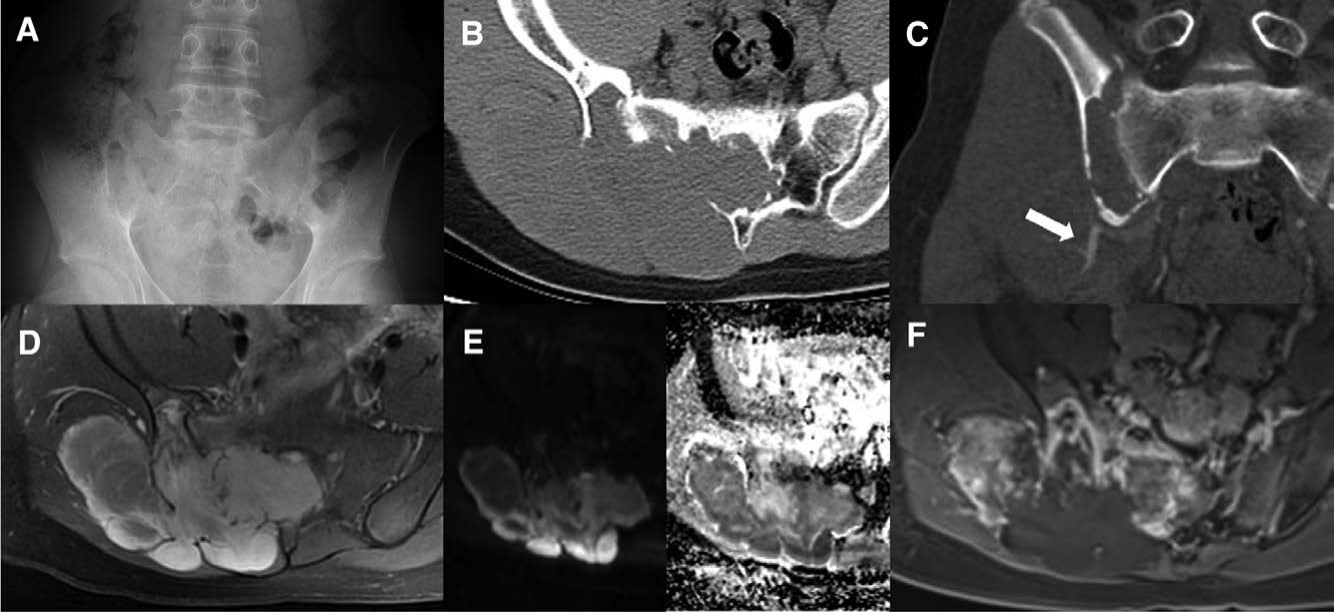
Preoperative X-ray, CT, and MRI of the SFT. (A) X-ray of lumbosacral vertebrae (anteroposterior view) manifested an expansive destruction of bone from S1 to S3 with ill-defined border, and the right sacroiliac joint space disappeared. (B) Contrast-enhanced abdominal CT scan showed a heterogeneously enhanced expansive tumor with the size of 82 × 77 × 115 mm occupying most of the pelvis with the expansion of sacral cannel and foramen. (C) Multiplanar reconstruction demonstrated that the blood supply was supplied by a branch of the right internal iliac artery (white arrow). (D) MRI exhibited a large tumor invading surrounding soft tissues, which demonstrated mixed signal on T2-weighted images with high signal dominated. (E) Diffusion weighted imaging and ADC maps demonstrated restricted diffusion with a reduction in ADC (ADC = 0.875 × 10–3 mm^2^/s). (F) Contrast-enhanced MR manifested obvious heterogeneous contrast enhancement. ADC = apparent diffusion coefficient, CT = computed tomography, MRI = magnetic resonance imaging, SFT = solitary fibrous tumors.
